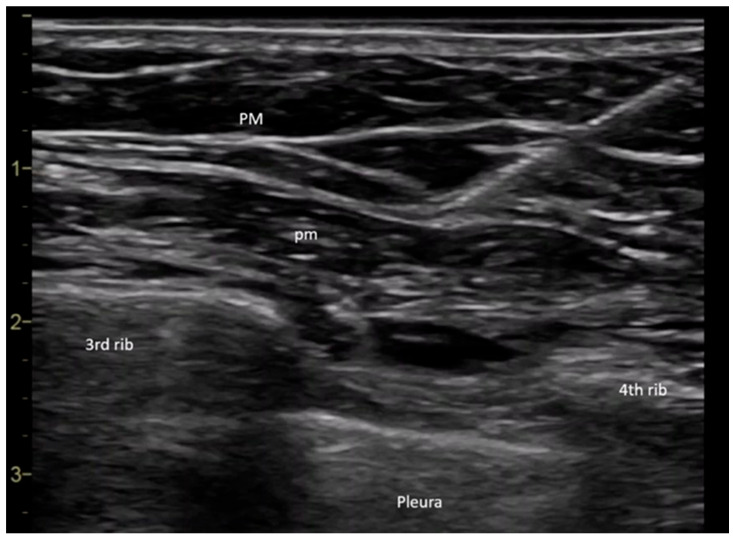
Ultrasonographic image showing the interfascial planes between the pectoralis major and minor muscles during local anesthetic injection (own archive). Legend: PM—pectoralis major muscle, pm—pectoralis minor muscle.
